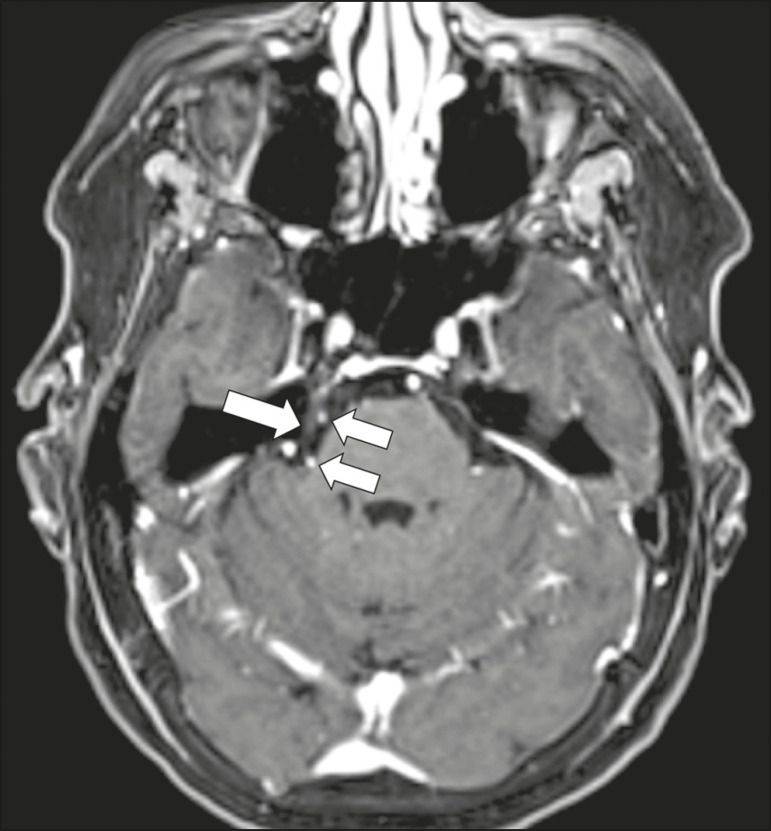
Neurovascular conflict. A 68-year-old female with right trigeminal neuralgia. Axial contrast-enhanced T1-weighted MRI scan showing a tortuous right superior cerebellar artery loop (medial arrows), tapering and displacing (laterally and inferiorly) the cisternal segment of the right trigeminal nerve (lateral arrow), including its origin at the pons.
